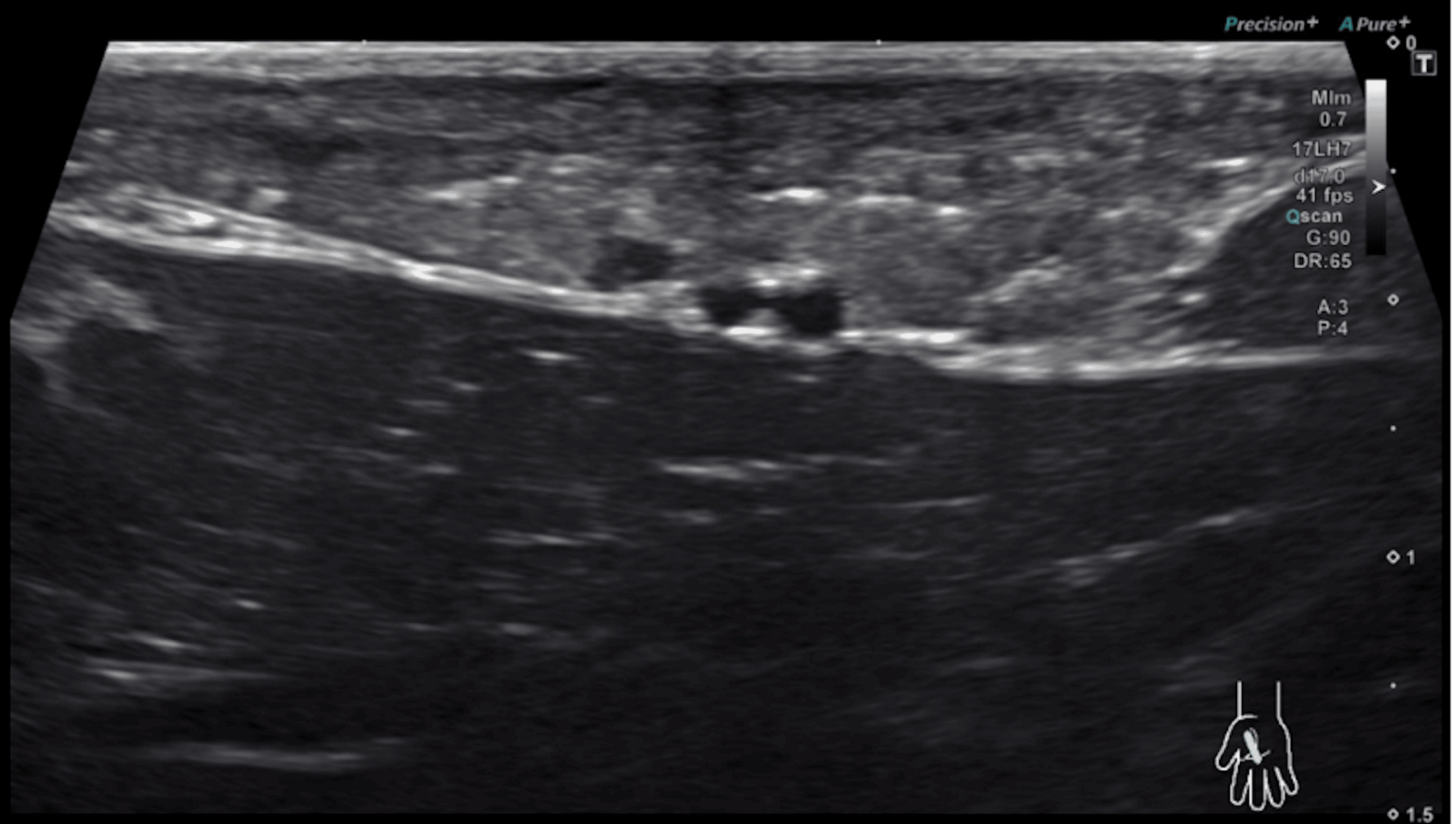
Ultrasound image of the palmar abnormality in longitudinal section This image demonstrates the hyperechoic fibrous thickening of the first web space consistent with fibrotic contracture and loss of normal soft-tissue architecture.
